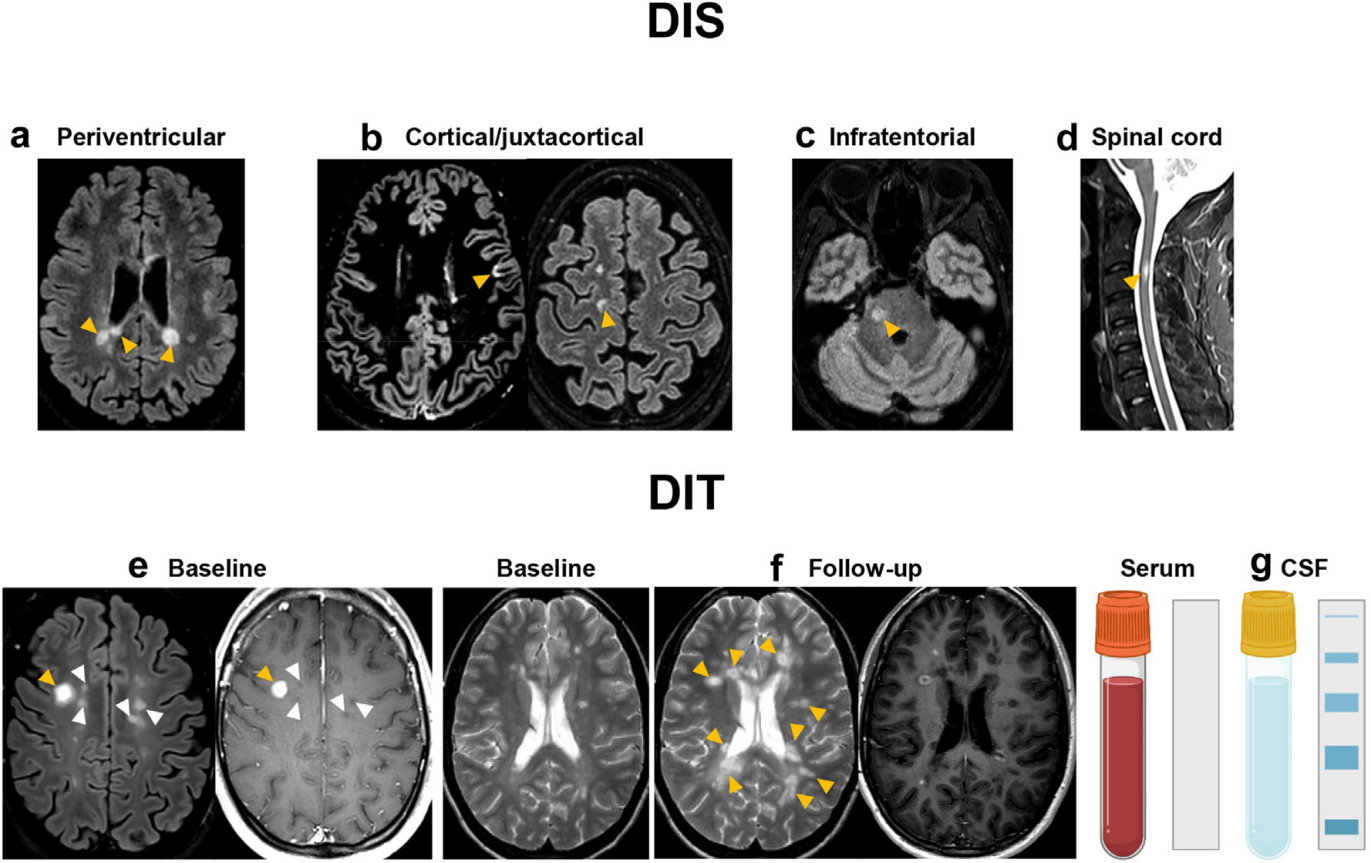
2017 McDonald criteria for demonstration of DIS and DIT in patients with a CIS suggestive of MS. Typical MRI examples (orange arrowheads) of (a) periventricular, (b) cortical/juxtacortical, (c) infratentorial and (d) spinal cord MS lesions. DIS can be demonstrated by ≥1 T_2_-hyperintense lesions in ≥2 of 4 typical areas of the central nervous system (i.e., periventricular, cortical/juxtacortical, infratentorial or spinal cord). DIT can be demonstrated by (e) a simultaneous presence of Gd-enhancing (orange arrowhead) and non-enhancing (white arrowheads) lesions at any time; (f) a new T_2_-hyperintense and/or Gd-enhancing lesion on follow-up MRI (orange arrowheads), with reference to a baseline scan, irrespective of the timing of the baseline MRI. For the definition of both DIS and DIT, the distinction between symptomatic and asymptomatic lesions has been removed in the 2017 revision of the McDonald criteria. (g) In patients with a typical CIS suggestive of MS fulfilling DIS criteria and with no better explanation for the clinical presentation, the demonstration of CSF-specific OCBs, i.e., not present in the serum but only in the CSF, substitutes for the requirement of fulfilling DIT, thus allowing a diagnosis of MS, even if the clinical and MRI findings do not meet the criteria for DIT. Abbreviations: CIS, clinically isolated syndrome; CSF, cerebrospinal fluid; DIS, dissemination in space; DIT, dissemination in time; Gd, gadolinium; MRI, magnetic resonance imaging; MS, multiple sclerosis.
